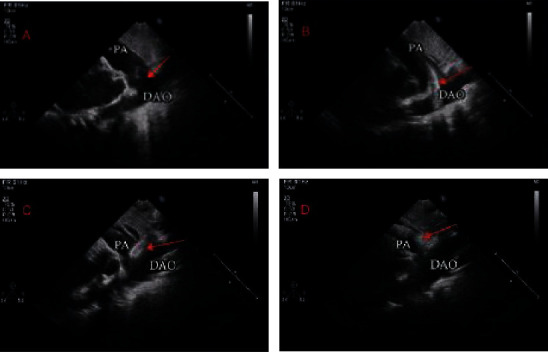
Transcatheter closure of a PDA under TTE guidance via femoral vein. (a) The PDA (arrow) was measured using the parasternal short-axis view. (b) The parasternal short-axis view showed that the guide wire (arrow) was located within the descending aorta. (c) Release of the aortal side disk of the occluder (arrow). (d) Release of the pulmonary side disk of the occluder (arrow). DAO = descending aorta; PA = pulmonary artery.
